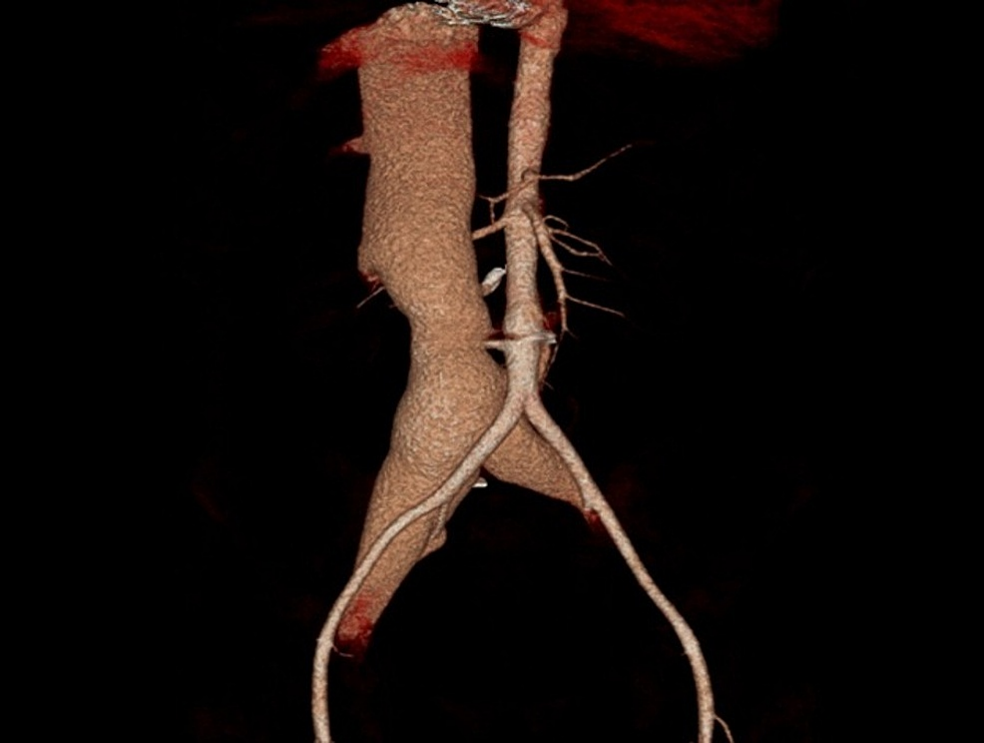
Computed tomography angiography (volume rendering) A communication between the aorta ( just above the aortic bifurcation) and the inferior vena cava. It shows the severely dilated inferior vena cava.
